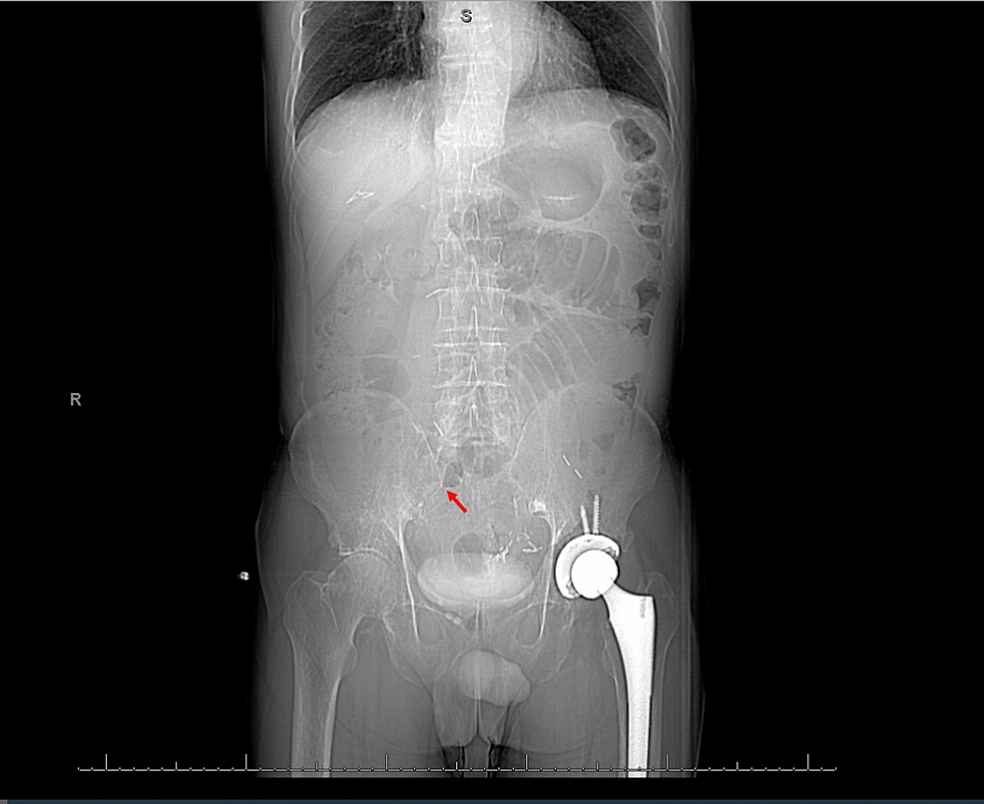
CT scan of the abdomen and pelvis without contrast (coronal view) demonstrating dilated loops of small bowel with a transition point near the terminal ileum (arrow), consistent with small bowel obstruction. CT: computed tomography
